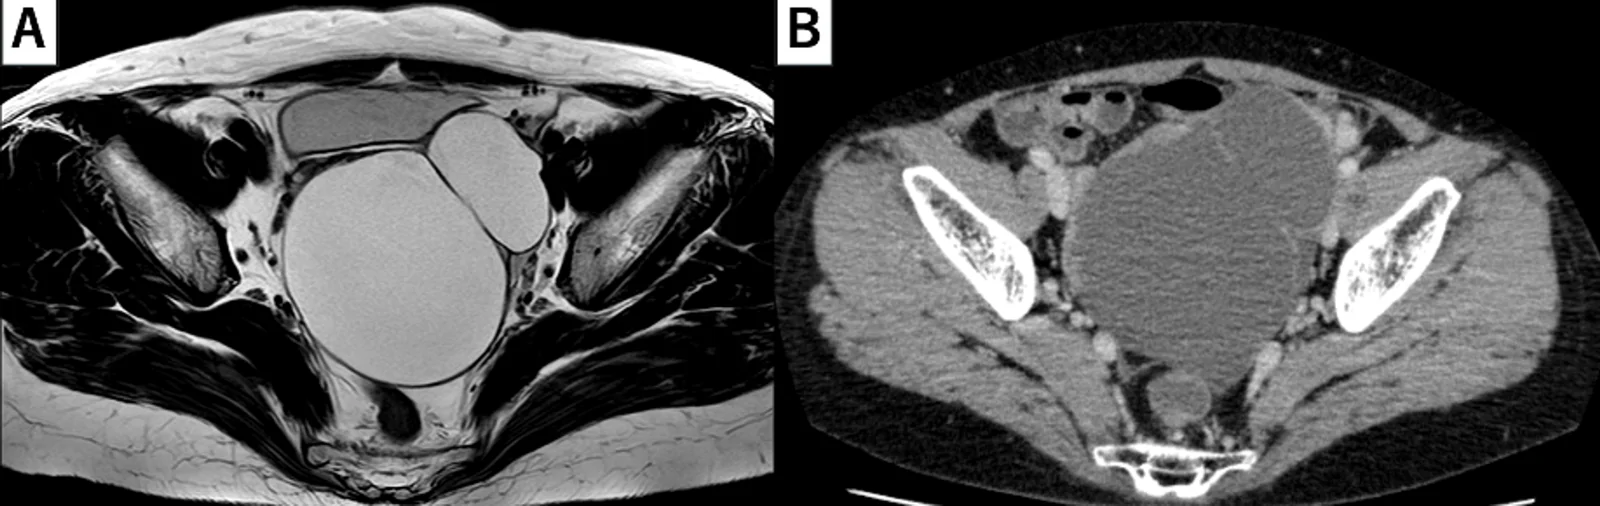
Radiologic findings. (A) Axial T2-weighted magnetic resonance imaging showed a large multilocular cystic tumor in the pelvis. (B) Contrast-enhanced computed tomography revealed no enhancement of the cyst wall or internal septa; there were no findings suggestive of malignancy.
